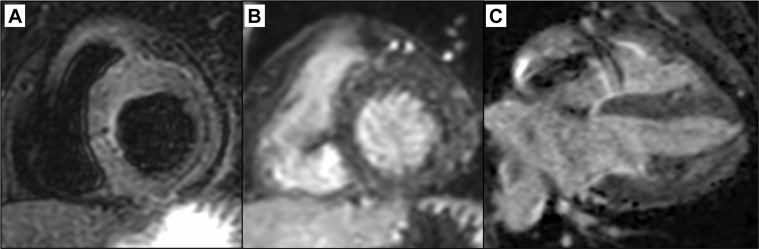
Cardiac Magnetic Resonance in the Chronic Phase (A) T2-weighted images revealed no hyperintense areas indicating myocardial edema. (B and C) There was no late gadolinium enhancement throughout the myocardium including conduction areas.
